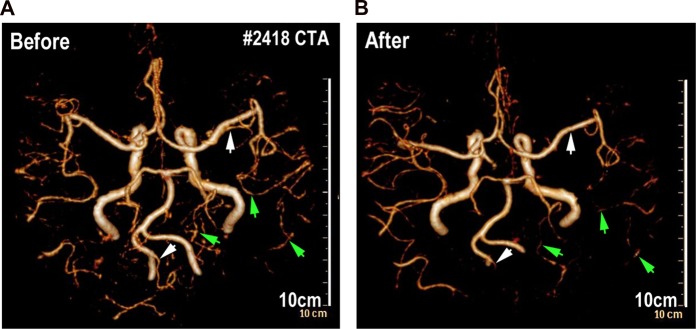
Whole brain radiations decrease the density of cerebral vessels in human patient. (A) CTA vascular reconstruction images depicting cerebral vascular of patients before whole-brain radiotherapy (left) and 4 months after whole-brain radiotherapy (right). The arrowheads indicate cerebral vascular before whole-brain radiotherapy (left) and after (right).
